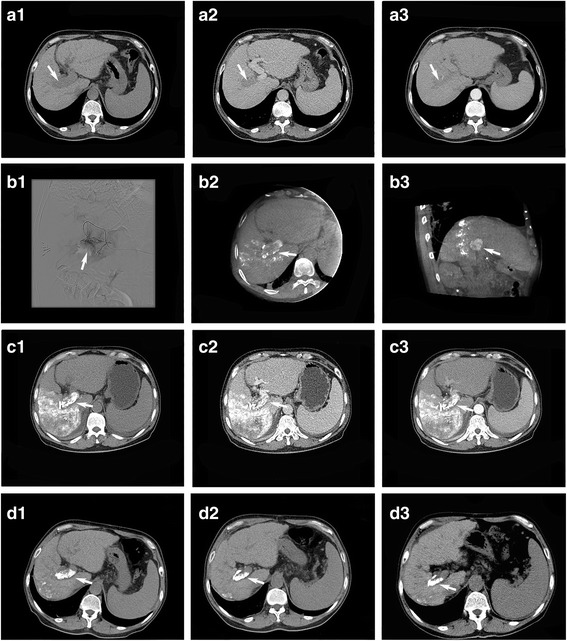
Intraarterial ethanol embolization with TACE in a 64-year-old male with HCC and PVTT (Vp3). (A1, A2) CT scan in the portal venous phase highlighting PVTT in the right portal vein (arrow) is shown; (A3) PVTT-feeding artery identified on CT. (B1) PVTT-feeding artery identified on DSA by superselective catheterization of the feeding artery using a microcatheter; (B2, B3) enhanced C-arm CT was performed to further confirm the PVTT-feeding artery; (C1-C3) axial CT showing lipiodol-ethanol mixture deposition within PVTT. (D1-D3) Follow-up images showing stable lipiodol-ethanol mixture deposition within PVTT at 3, 6, and 12 months after the operation
